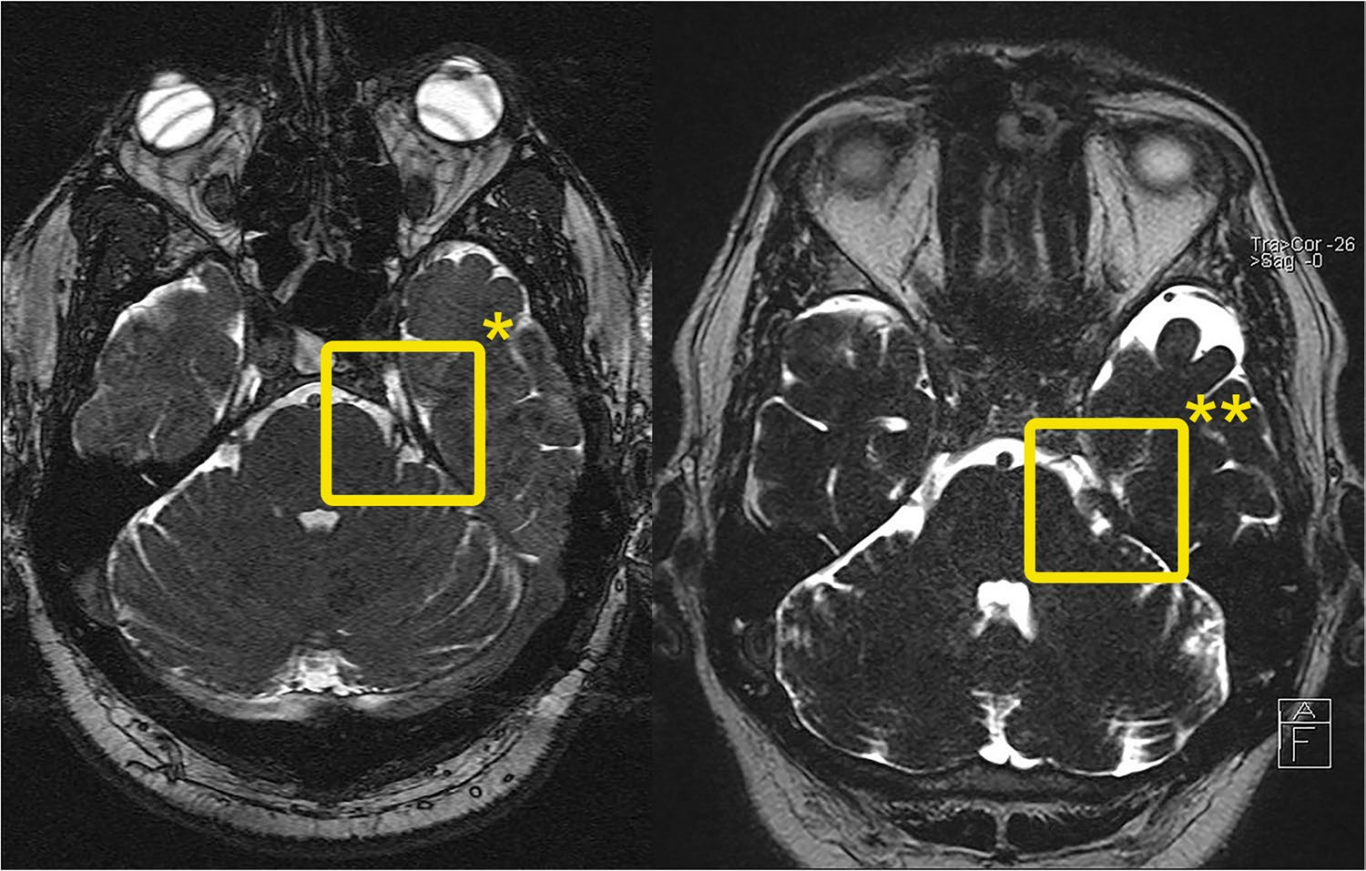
Male patient in his 5th decade of life with classical TGN in the 2nd and 3rd divisions of the left TGN. Following a unsuccessful first MVD at another institution, the patient underwent four subsequent radiofrequency thermocoagulations of the trigeminal ganglion at our clinic resulting in short periods of pain improvement of approximately 1 year and pain reduction with every intervention. Due to these short periods, classic symptoms and a still existing NVC in MRI (*); a second MVD was performed which revealed insufficient decompression and resulted in a BNI pain intensity score of I. Unfortunately, pain recurrence occurred after 2 years in the first division of the left TGN. MRI () showed the TGN root surrounded by Teflon™ and the SCA with a further close relationship. After a long consultation, the patient requested another exploration. During the surgical procedure, the nerve-Teflon™ convolute was left in place, the adjacent SCA was sharply dissected away and isolated again, which resulted in a current BNI pain intensity score of I
